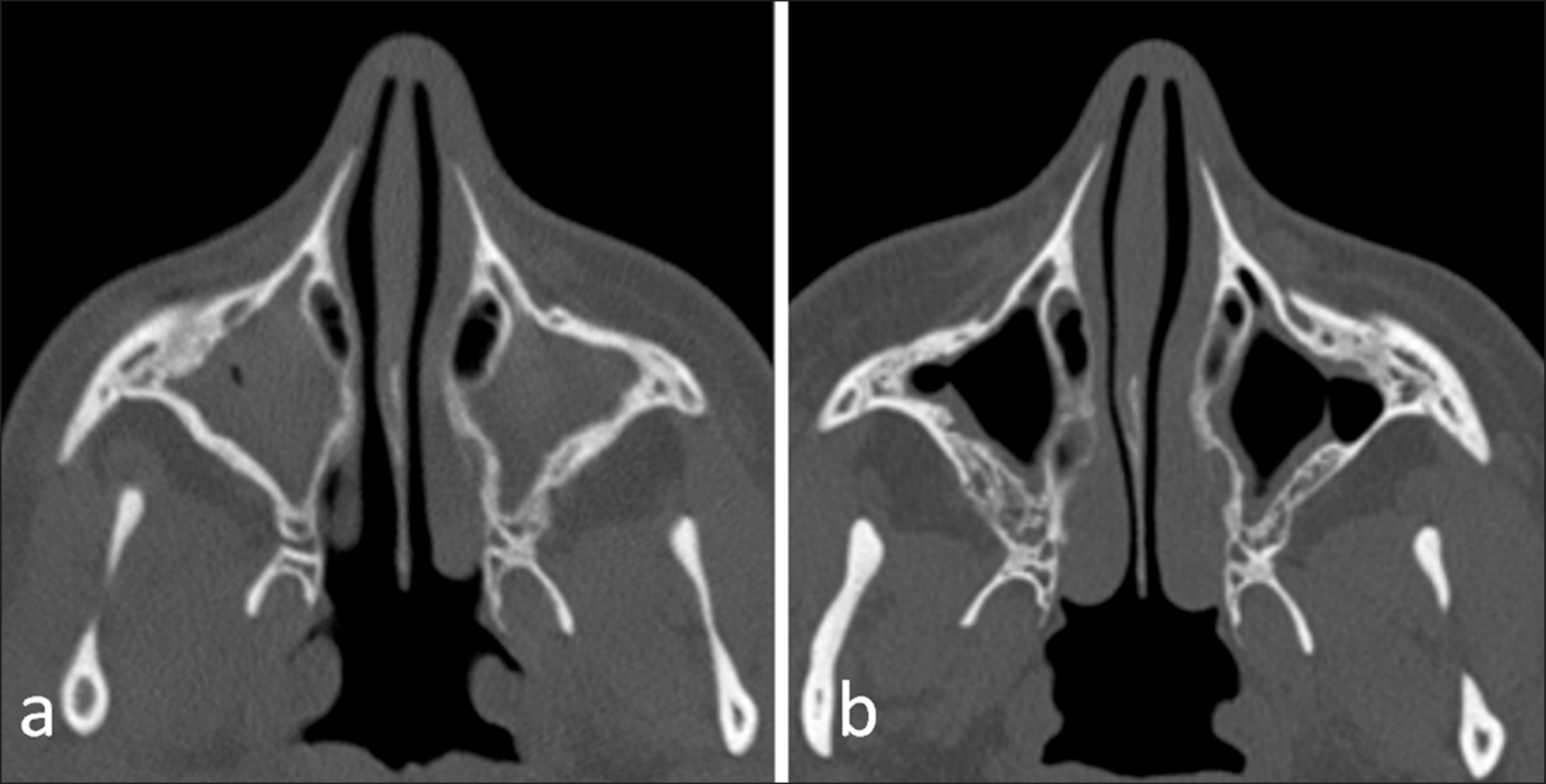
Axial sinus computed tomography images from a cystic fibrosis patient with chronic rhinosinusitis before (a) and after (b) one year of modulating therapy.
